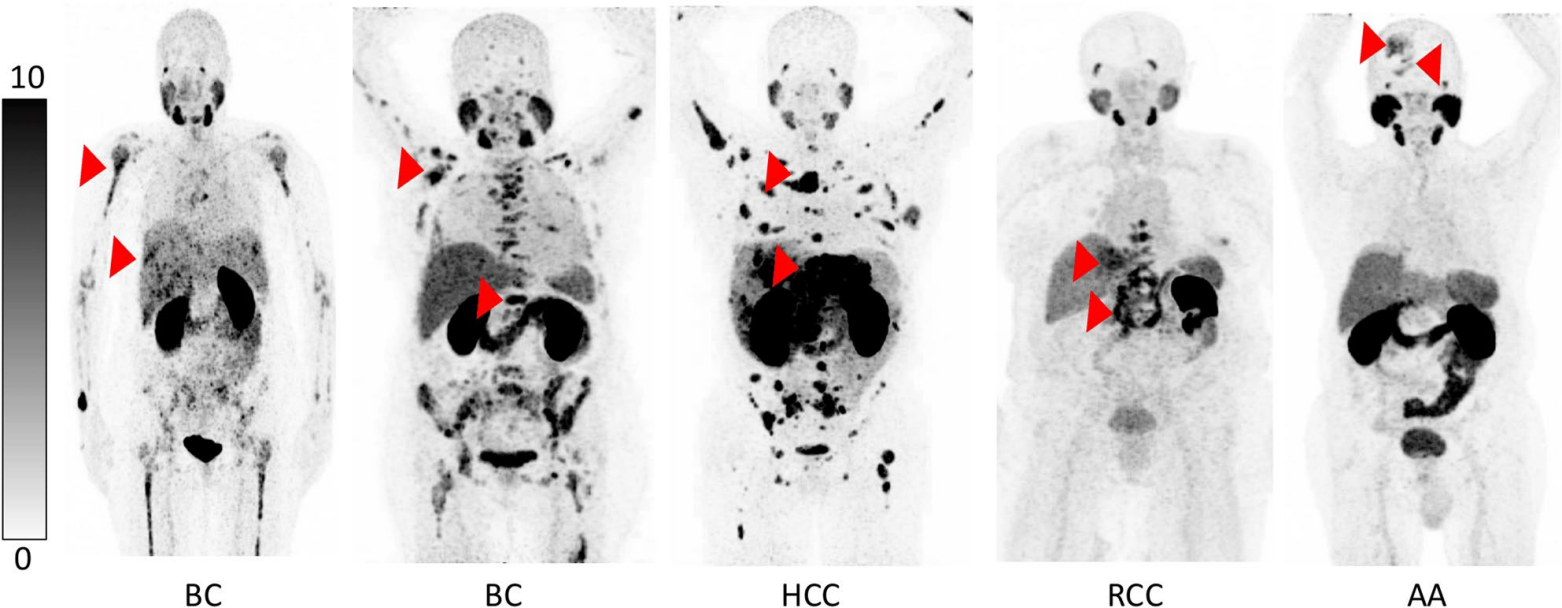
Maximum intensity projections (MIP) of screening [^68^Ga]Ga-PSMA-11 PET/CT in non-PCa patients. Red arrows point to exemplary tumor lesions. BC, breast cancer; HCC, hepatocellular carcinoma; RCC, renal cell carcinoma; AA, anaplastic astrocytoma
